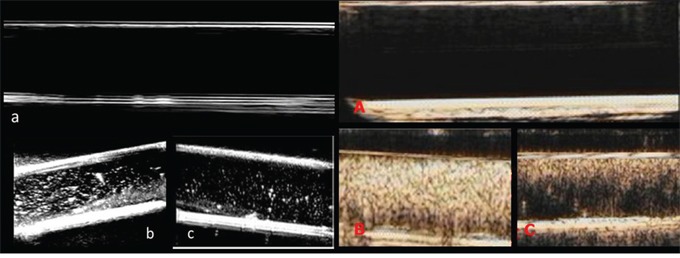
Two-dimensional ultrasonic imaging and ultrasound contrast enhanced imaging of UCAs *in vitro* Two-dimensional ultrasonic images: a. a tube filled with degassed aqueous, b. SonoVue in degassed aqueous. c. NPs in degassed aqueous. Ultrasound contrast enhanced images: A. a tube filled with degassed aqueous, B. SonoVue in degassed aqueous. C. NPs in degassed aqueous.
